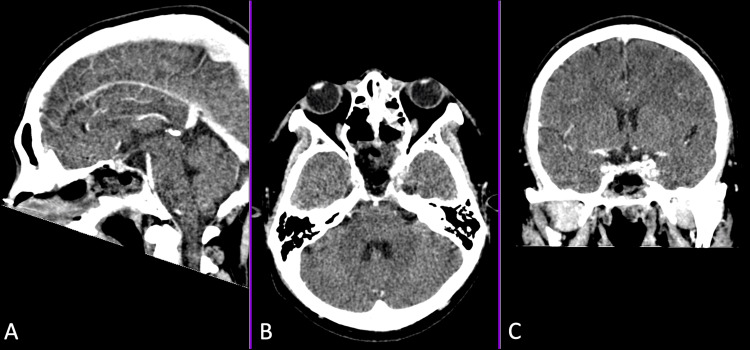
Postoperative head CT with contrast showed a slight decrease in the bulk of the clival mass to 3.2 x 3.0 x 1.0 cm (AP x W x CC). (A) Sagittal view, (B) Axial view, and (C) Coronal view.
